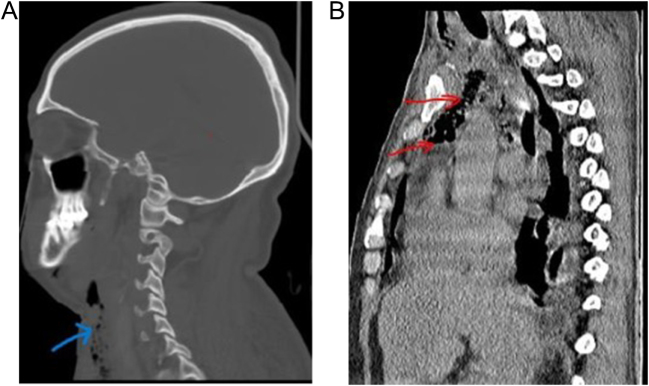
(A and B). CT scan sagittal view showing the extensive pretracheal subcutaneous tissue gas collection (A) extending downward into the superior mediastinum (B).
